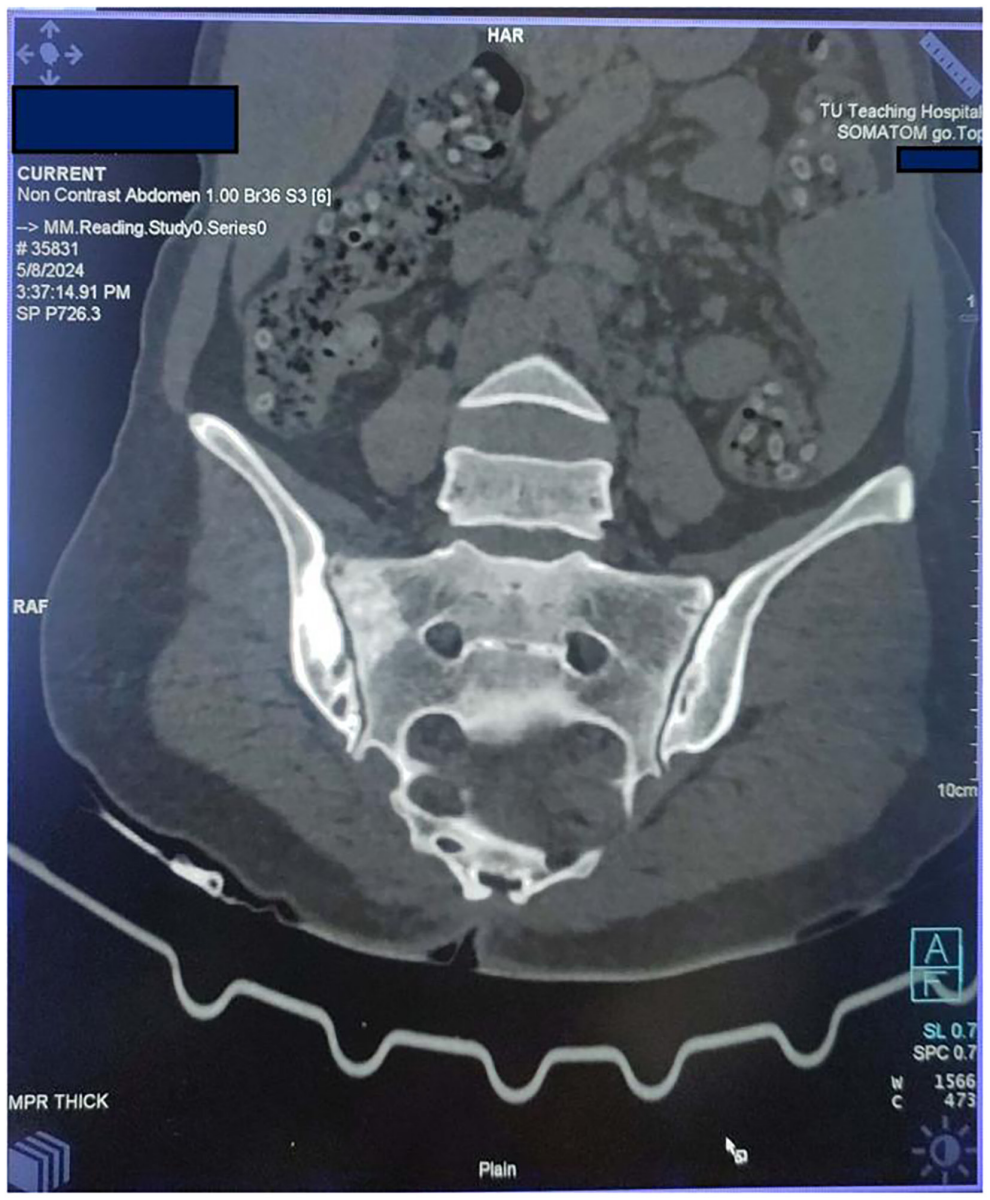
Axial bone window CT scan showing sacroiliitis of right sacroiliac joint.
